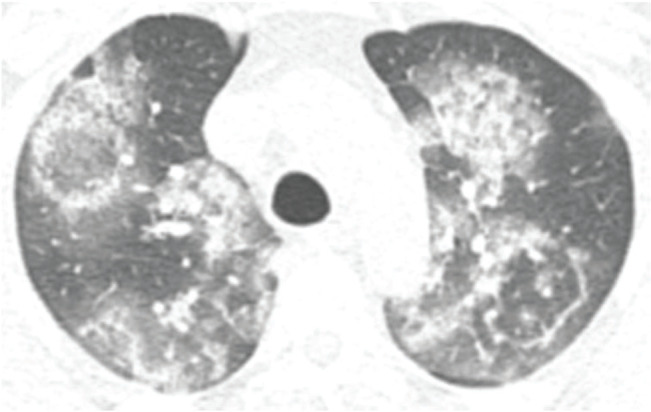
Typical findings of COVID-19. Axial CT image shows GGO areas surrounded by rings of consolidation (reversed halo sign), complete and incomplete.
